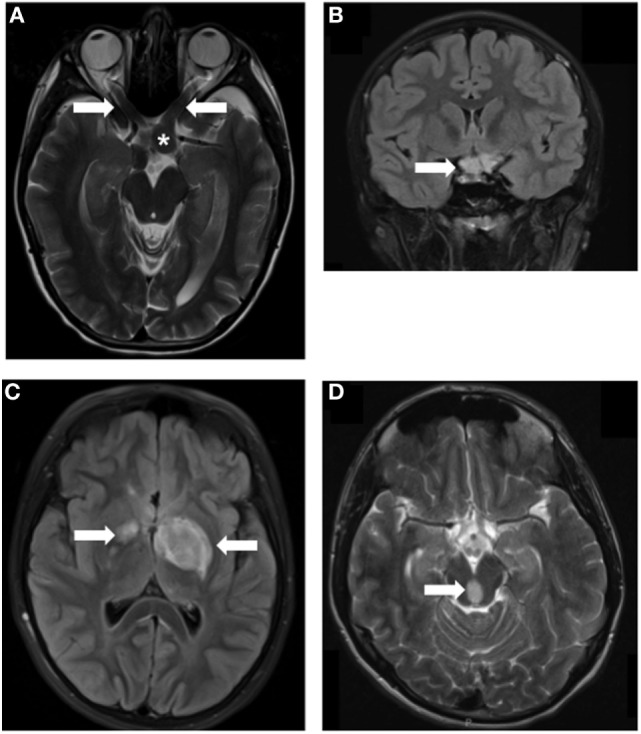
MRI images of Neurofibromatosis type 1 (NF1)-associated low-grade glioma (LGG). LGGs (arrows) developing in children with NF1 at various locations, including optic nerves (A), asterisk indicates the chiasm, chiasm (B), thalamus (C), and brainstem (D). Arrows denote the location of the tumors.
